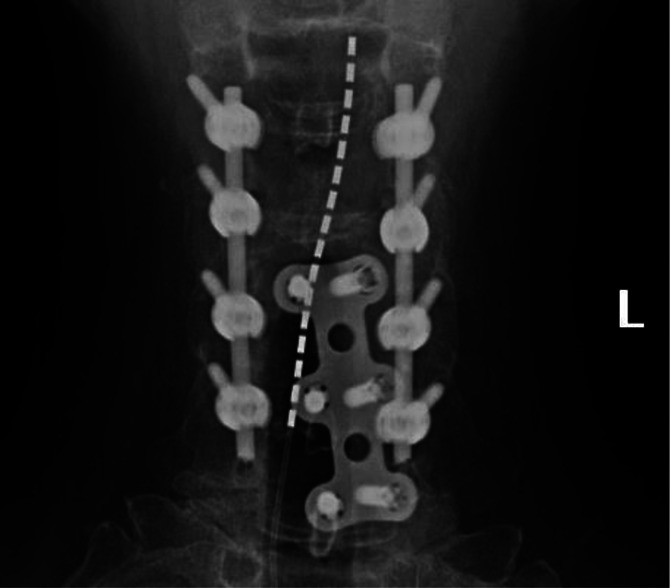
Anteroposterior view x-ray of patient's cervical spine obtained through fluoroscopy. Spinal cord stimulator lead shown to be placed at the bottom of C1 vertebral body with anterior and posterior hardware present.
